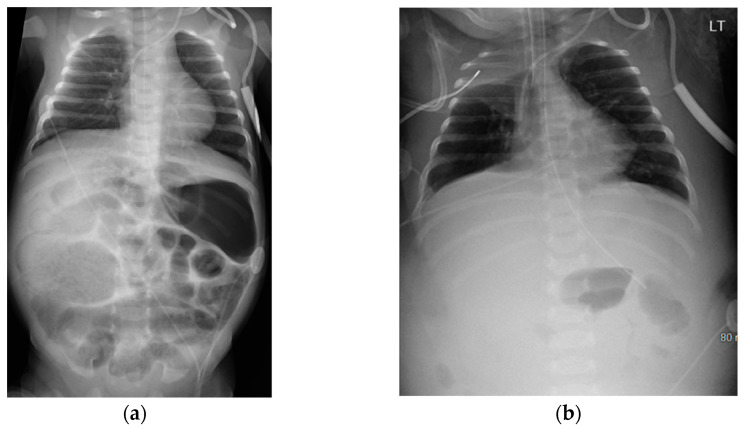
Abdominal X-ray on Day 41 and Day 50 suggesting recurrent necrotizing enterocolitis; (a) Day 41 abdominal X-ray revealing pneumatosis with portal venous gas; (b) Day 50 Abdominal X-ray revealing abdominal distension with featureless bowlet loops.
